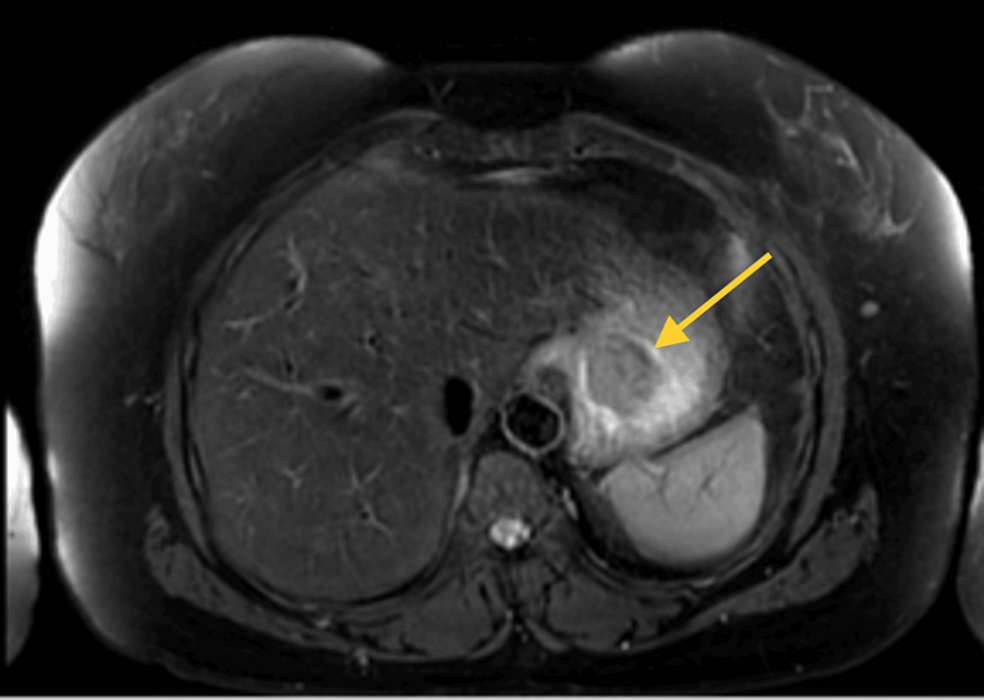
An abdominal MRI revealed the presence of a gastric fundus mass, indicative of a GIST (arrow). GIST: Gastrointestinal stromal tumor
